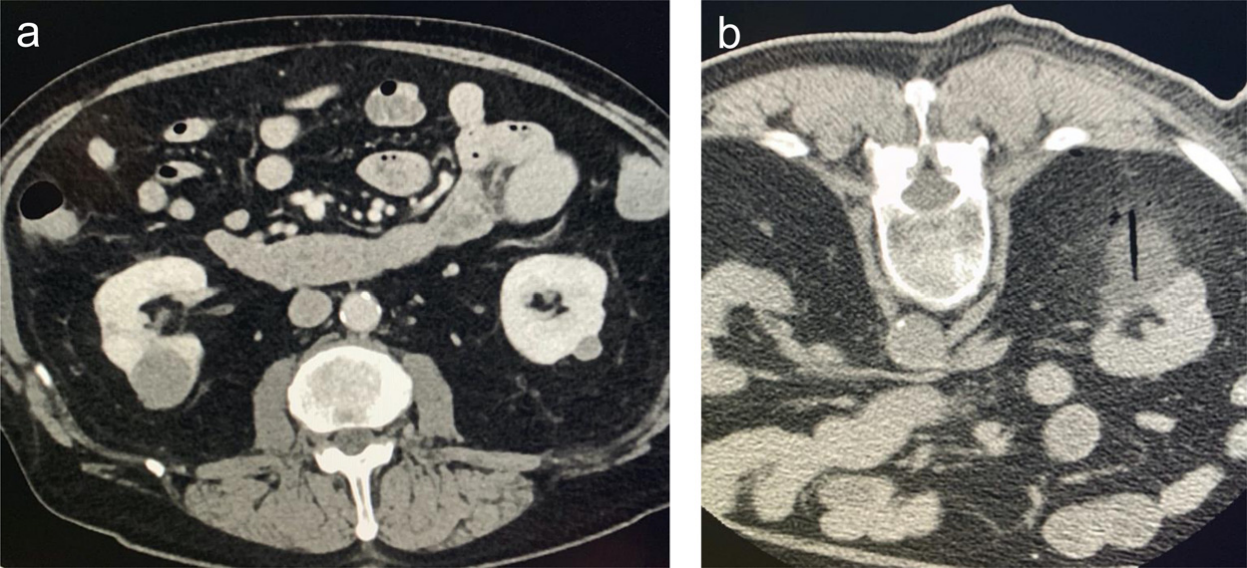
(A) Axial contrast enhanced CT image in nephrographic phase demonstrating a 2.7 cm, homogeneously enhancing renal mass in the right kidney. (B) Cross-sectional image demonstrating cryosection probe in place during second freeze cycle that shows an ice ball covering the treated mass with no significant perirenal hematoma.
